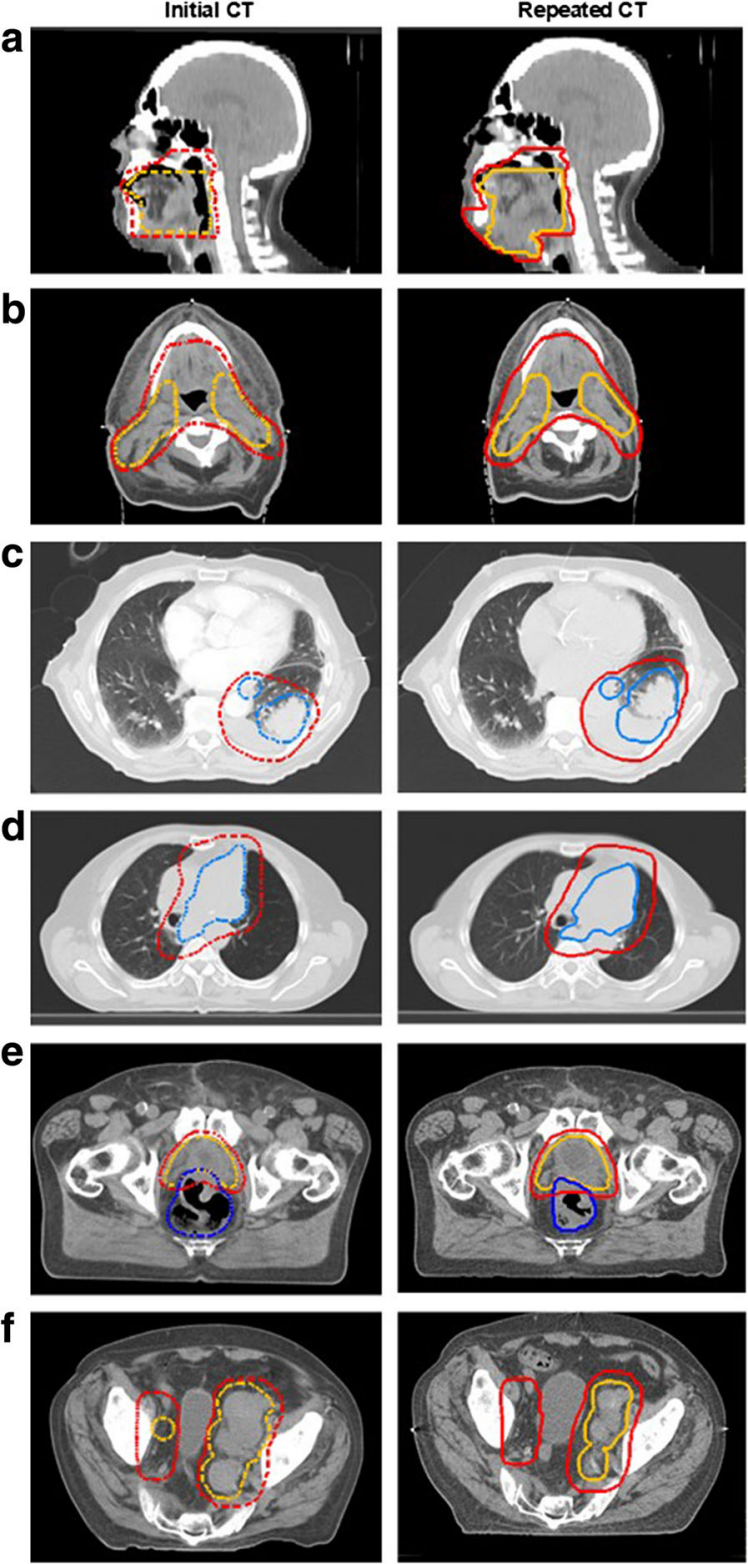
Example cases of anatomical changes during the treatment course for different entities. PTVs are shown in the initial CT (dashed lines, left side) and adapted PTVs in the repeated CT (solid lines, right side): a Head and neck case of tongue cancer (left) with tumor progress (right). The PTVs receiving 56,1 Gy and 66 Gy are highlighted in red and orange, respectively. b Head and neck case and CUP syndrome (left) with volume change due to edema reduction (right). PTVs receiving 59,4 Gy and 66 Gy contours are highlighted in red and orange, respectively. c Thorax case with lung cancer (left) and a growing pleural effusion (right). PTV receiving 50,4 Gy and 61,6 Gy are highlighted in red and blue, respectively. d Thorax case with lung cancer (left) and a mediastinal shift (right). PTVs receiving 50,4 Gy and 61,6 Gy are highlighted in red and blue, respectively. e Pelvis case with prostate cancer (left) and change in rectal volume (right). PTVs receiving 45,9 Gy and 56,7 Gy are highlighted in red and orange, respectively. f Pelvis case with prostate cancer and irradiation of bone and lymphatic metastasis (left) and decrease in lymph node size (right). PTVs receiving 40 Gy and 50 Gy are highlighted in red and orange, respectively
